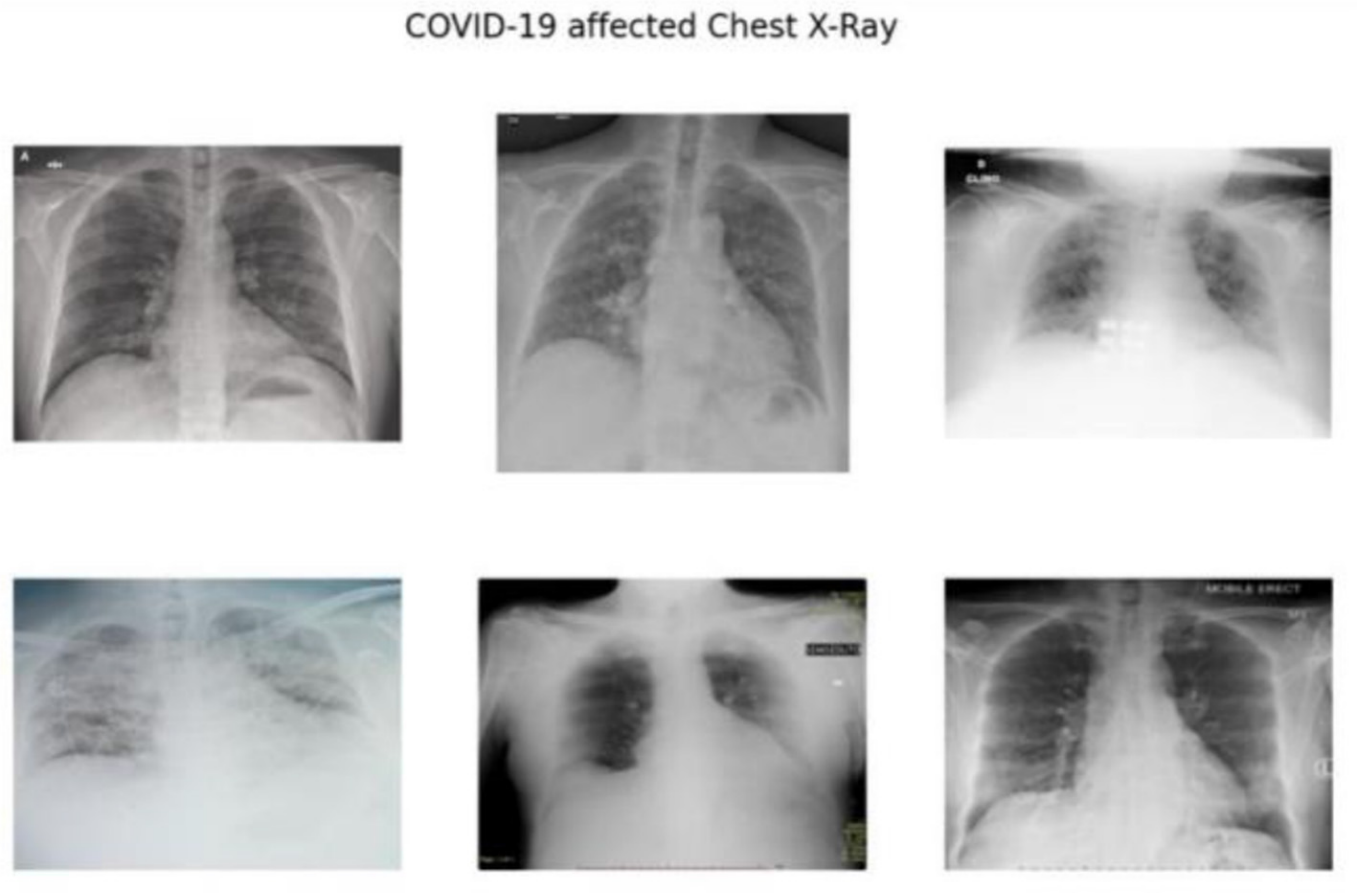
Chest X-ray COVID-19 image samples evaluated using the Kaggle database.
